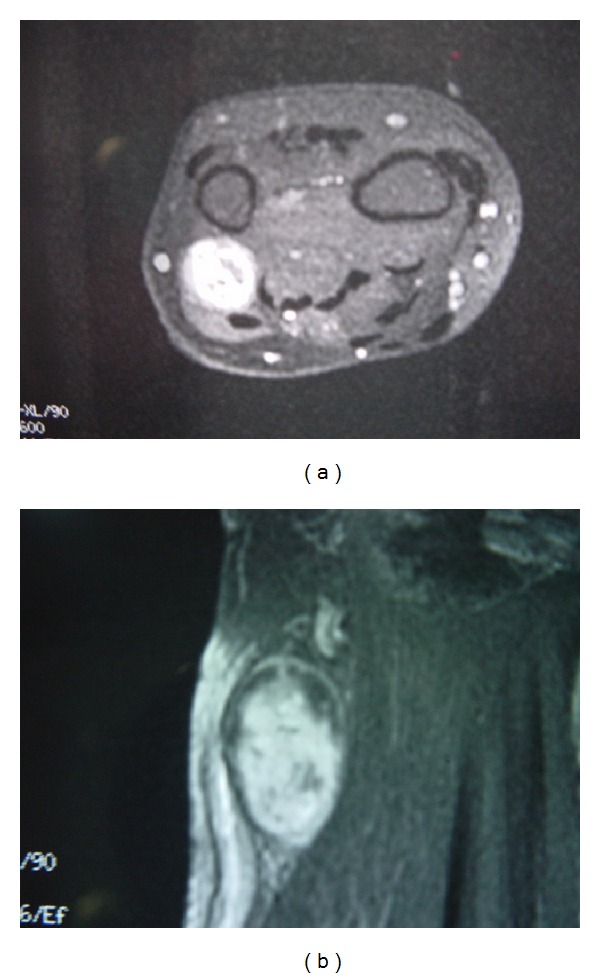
MRI showing schwannoma involving dorsal cutaneous branch of ulnar nerve, while the presence of fascicular involvement is not predictable in preoperative MRI.
